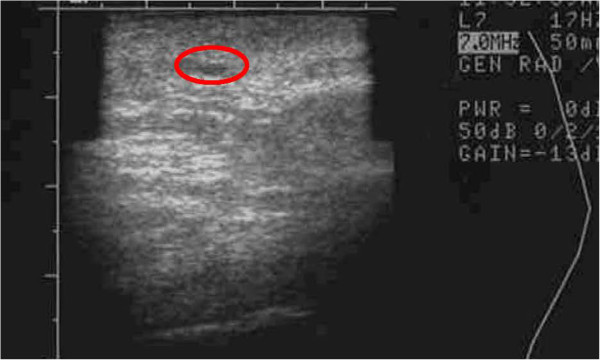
Ultrasound image of right hand (DEP-SVF treated) coronal view of thenar web space in a 69-year-old female 3 months post lipotransfer. Oil cyst seen in deeper dermis (red circle).
